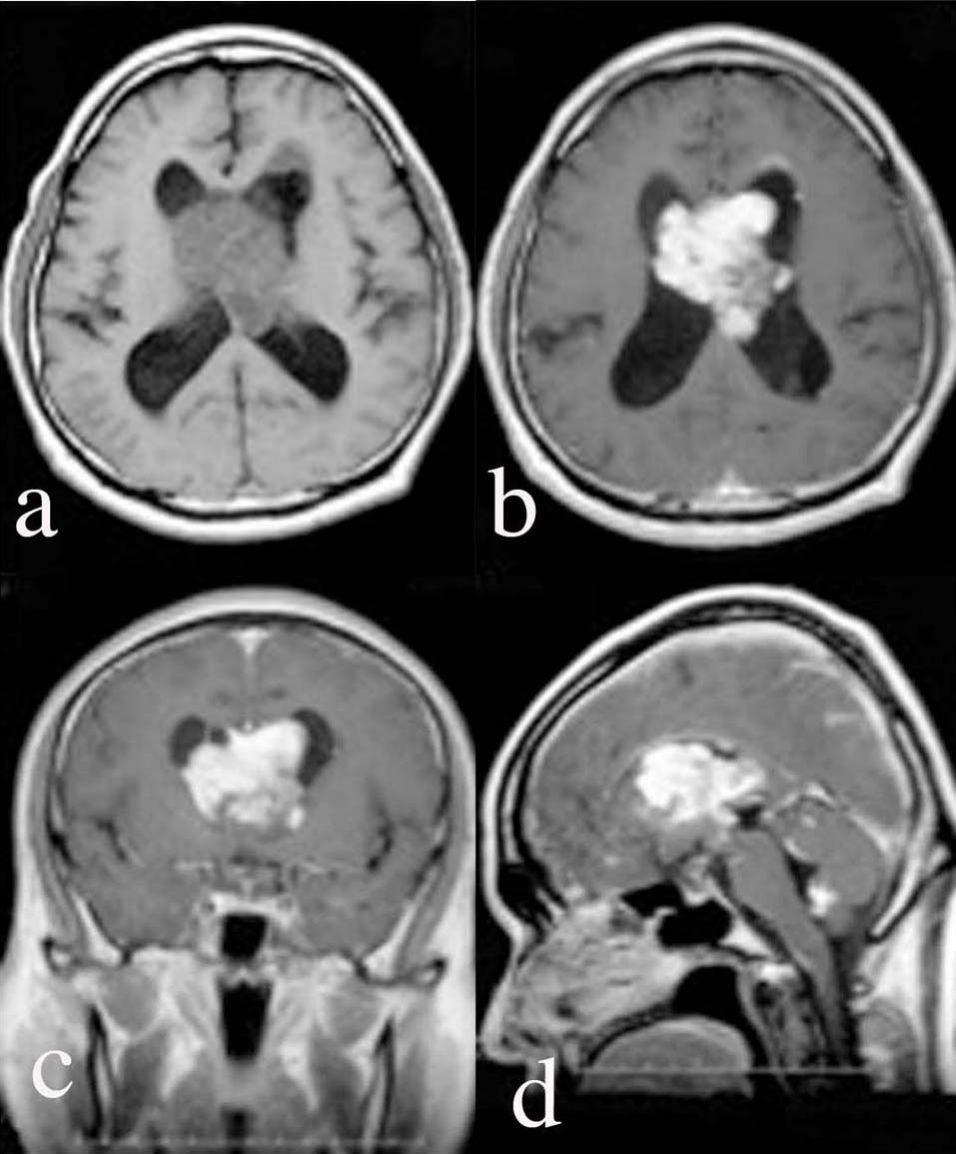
Magnetic resonance imaging (MRI) on admission. MRI [a: T1 plain-weighted image, b-d: Gadolinium-diethylenetriaminepentaacetic acid (Gd-DTPA)-enhanced images] scans showed a mass lesion in the lateral ventricles, the third ventricle, and the fourth ventricle. The mass lesion was enhanced with Gd-DTPA.
